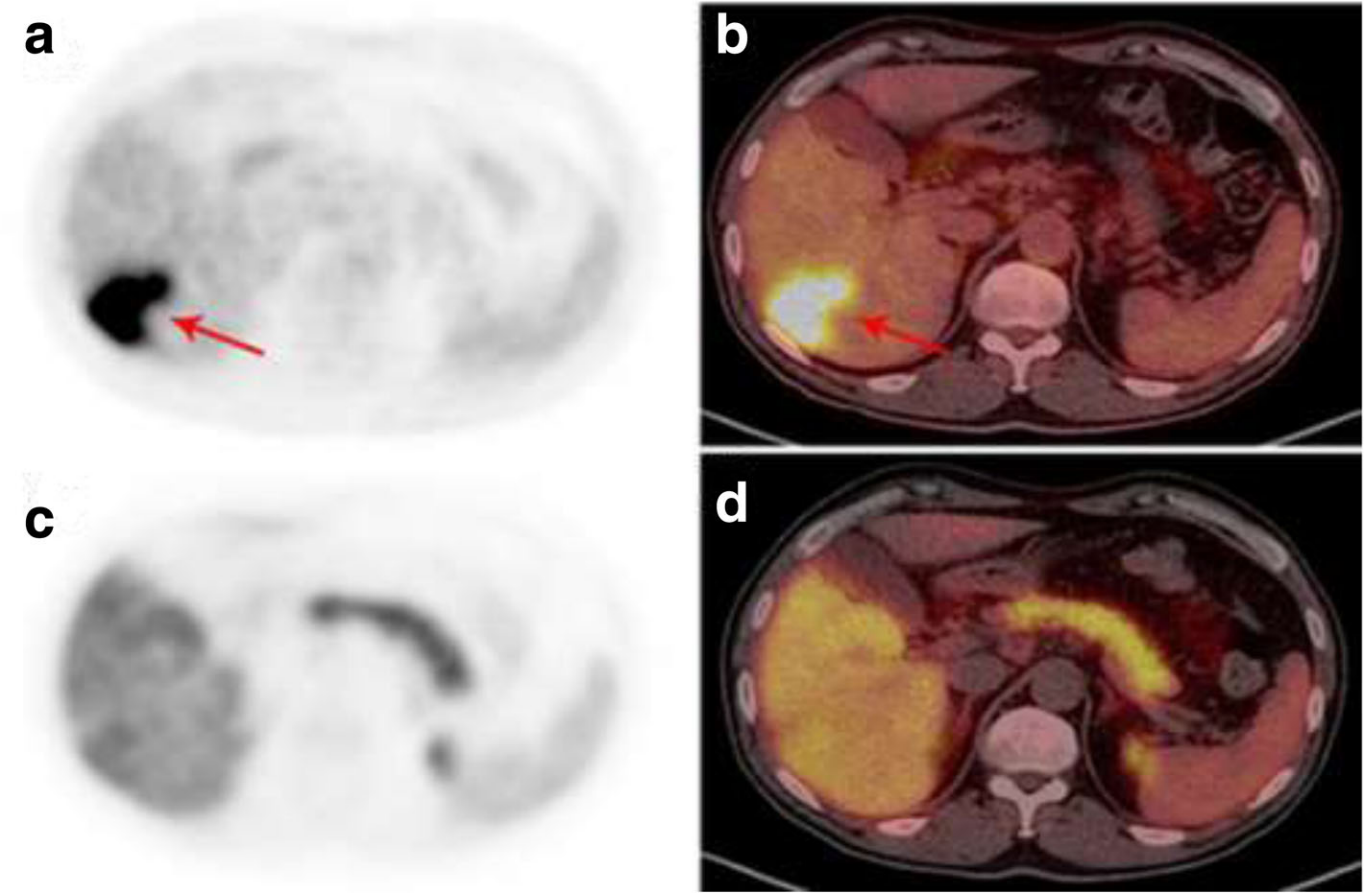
PET/CT with 18F–FDG and 18F–FCh in low-differentiated hepatocellular carcinoma. Patient (a). Low-differentiated hepatocellular carcinoma. Images of axial projections of PET (a, c) and combined images of PET/CT (b, d) with 18F–FDG (a, b) and with 18F–FCh (c, d). With PET/CT with 18F–FDG in the right lobe of the liver, the focus of pathological accumulation of the radiopharmaceutical agent (red arrows) is visualized. With PET/CT with 18F–FCh, the pathological accumulation of 18F–FCh in the liver is not determined
